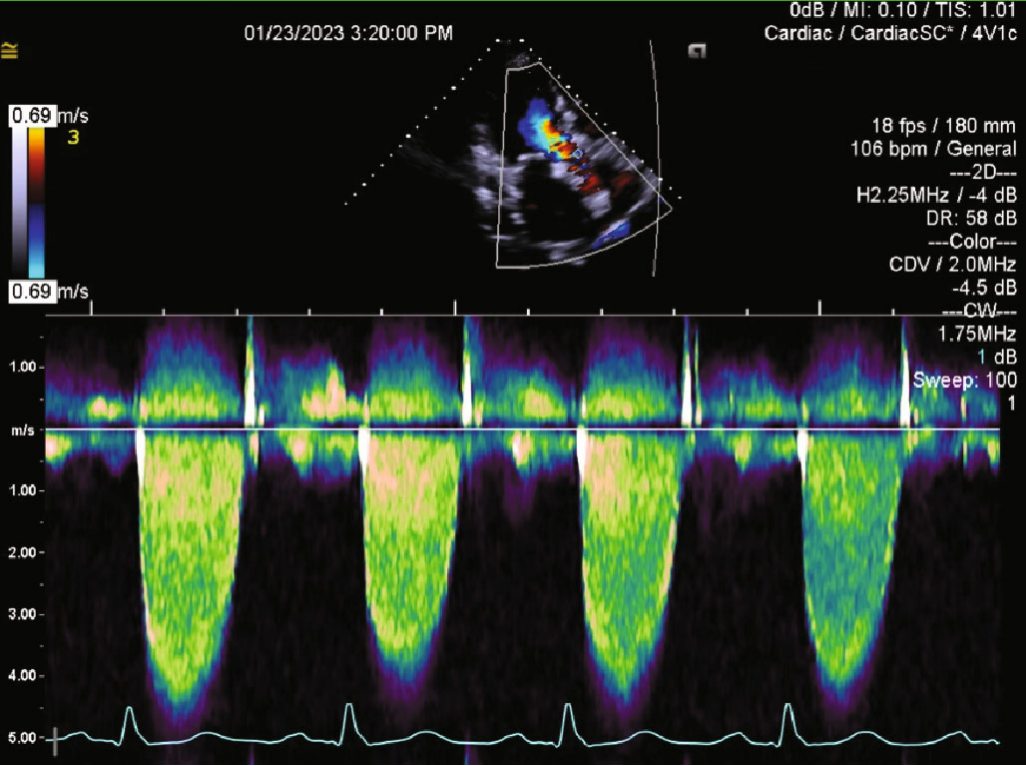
Continuous wave Doppler in the apical three-chamber view by transthoracic echocardiography. Mean transvalvular gradient of 51 mm Hg, peak prosthetic aortic jet velocity of 4.45 m/s, Doppler velocity index (DVI) of 0.20, and acceleration time of 104.25 ms.
